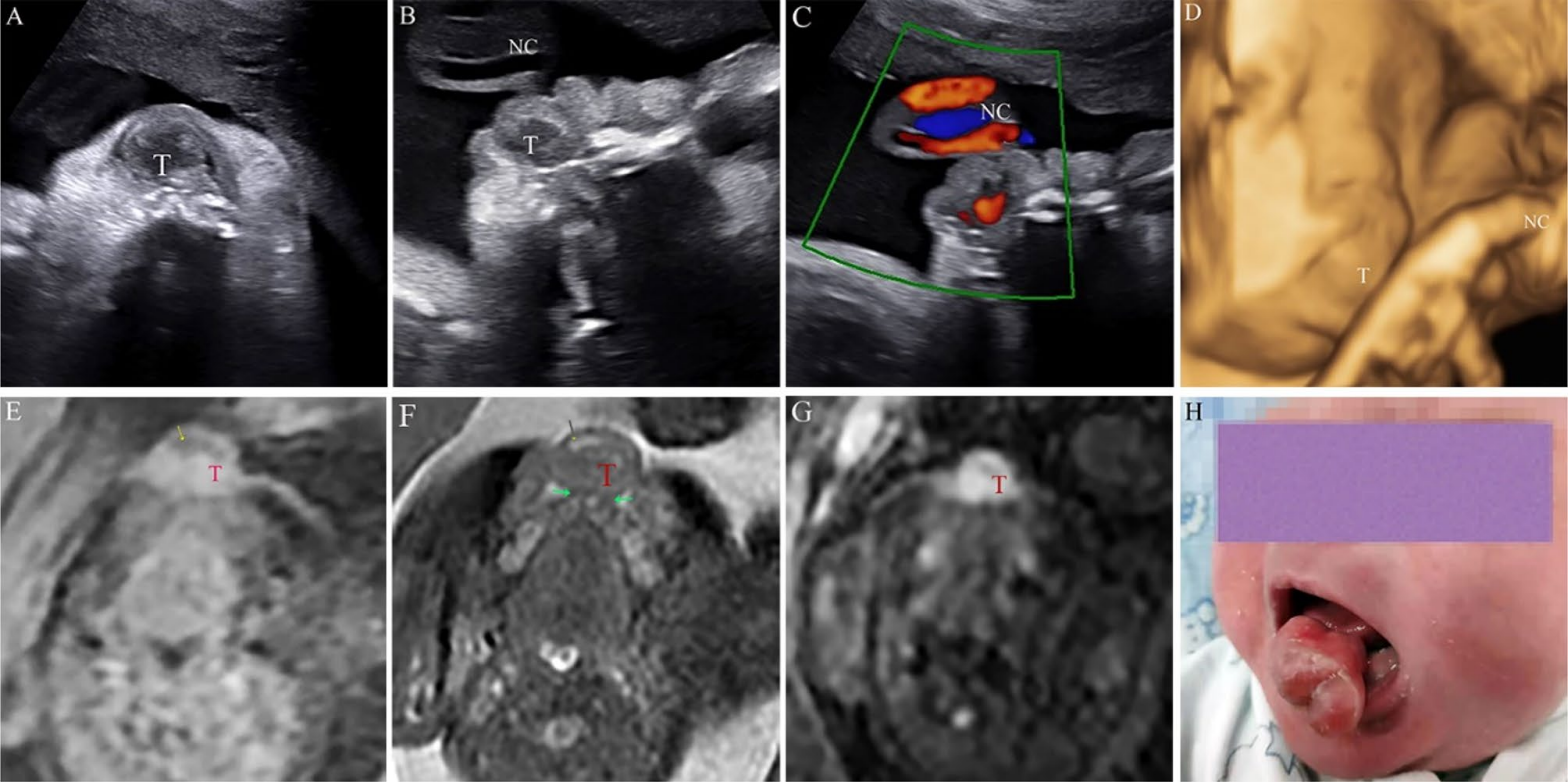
A congenital epulis at 34 weeks of gestational age. (A–B) On prenatal ultrasonic imaging, echography imaging revealed a low uneven echo mass (T) in the oral cavity that is lobulated with clear boundaries, an irregular shape. (C) Color Doppler ultrasound of blood flow showed abundant blood flow signals inside the mass, and nuchal cord (NC) was also shown. (D) Three-dimensional ultrasound of fetus, and was outward. (E) On T1WI, the lesion was with isointense signal and the peripheral part displayed a hypointense signal (yellow arrow). (F) On T2-weighted fat suppression image, the fat tissue was not detected while the mass was closely associated with the mandibular tooth bud (green arrow). (G) DWI image showed hyper-intensity in solid lesions of tumor tissues. (H) An image of the newborn.
